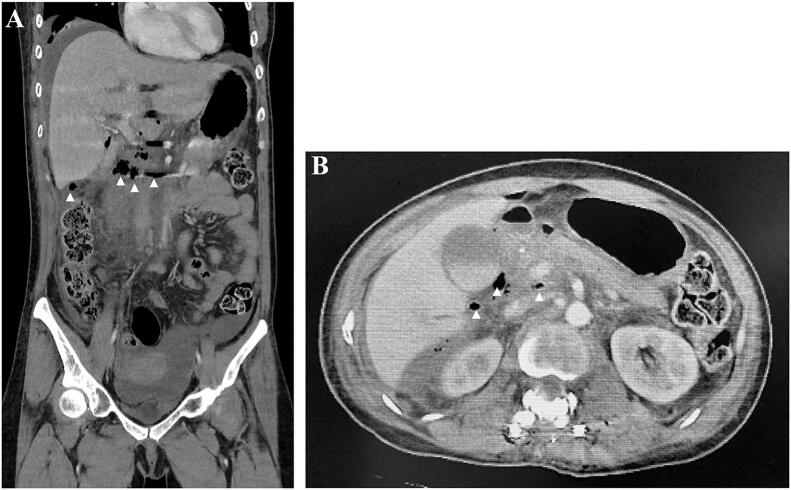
The contrast-enhanced chest and abdominal CT scans revealed free air surrounding the duodenum and liver, along with fat stranding and fluid accumulation (arrow heads) (A, B).
